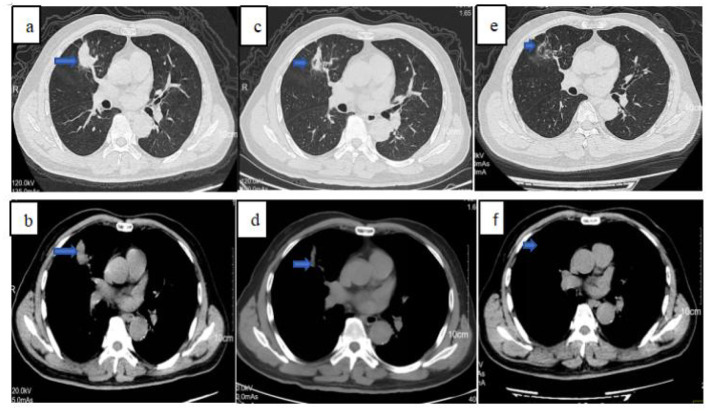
Outpatient follow-up chest CT results. Outpatient chest CT re-examination on December 27, 2025 (a, b), January 24, 2026 (c, d) and February 27, 2026 (e, f).
